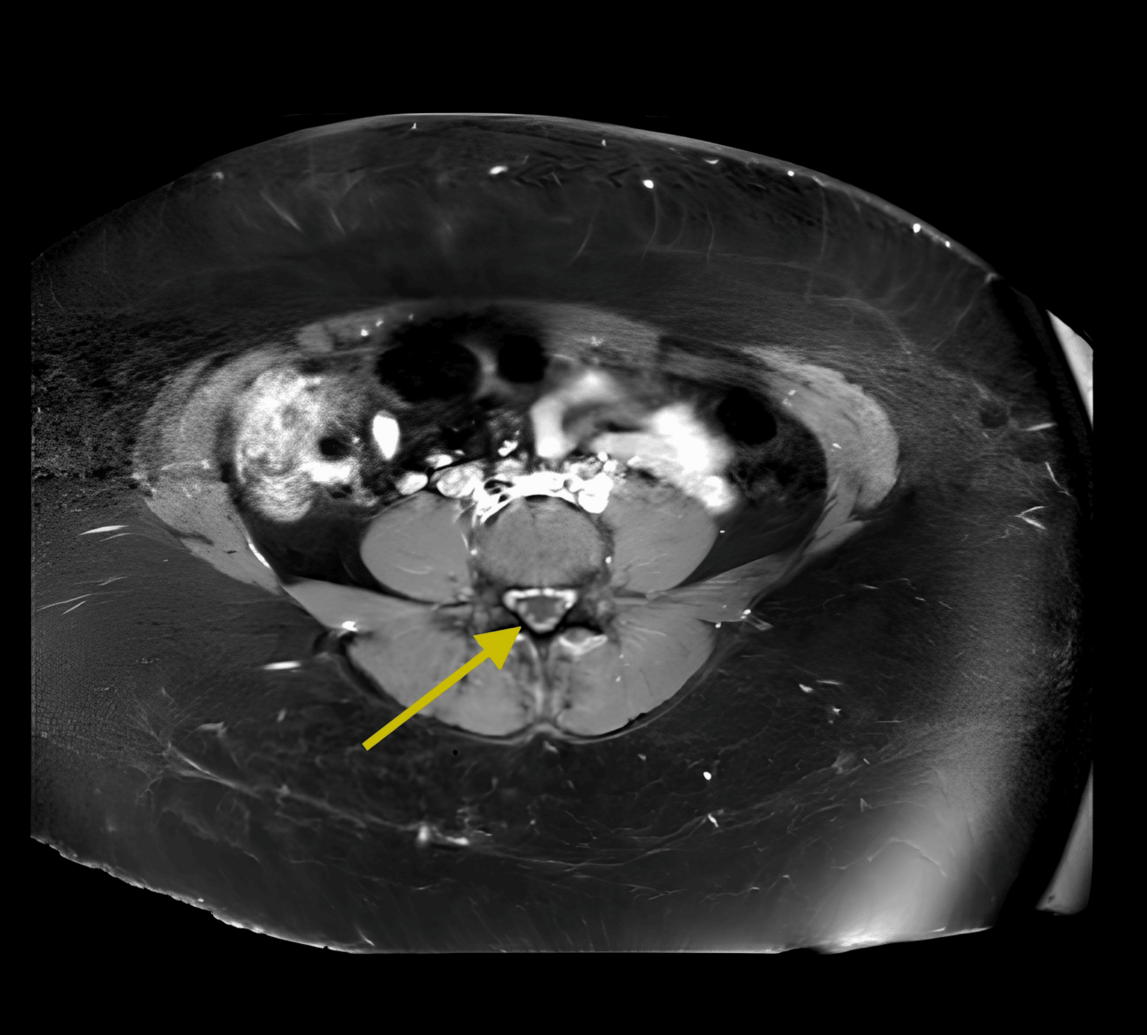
Contrast-enhanced MRI of the lumbosacral spine demonstrating diffuse enhancement of the cauda equina nerve roots, consistent with inflammatory polyradiculopathy T1-weighted image with gadolinium contrast showing enhancement of the cauda equina nerve roots.
